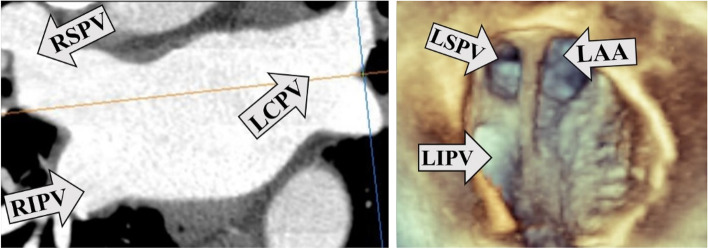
LCPV imaging. 4a left: LCPV detected by CCT in 2D longitudinal plane. 4b right: the same LCPV is not obvious on 3DTOE (full volume image, en face display). *LCPV* (Left common PV), *CCT* (Cardiac computed tomography), *2D* (Two-dimensional), *3DTOE* (Three-dimensional transesophageal echocardiography), *LSPV* (Left superior PV), *LIPV* (Left inferior PV), *LAA* (Left atrial appendage), *RSPV* (Right superior PV), *RIPV* (Right inferior PV)
